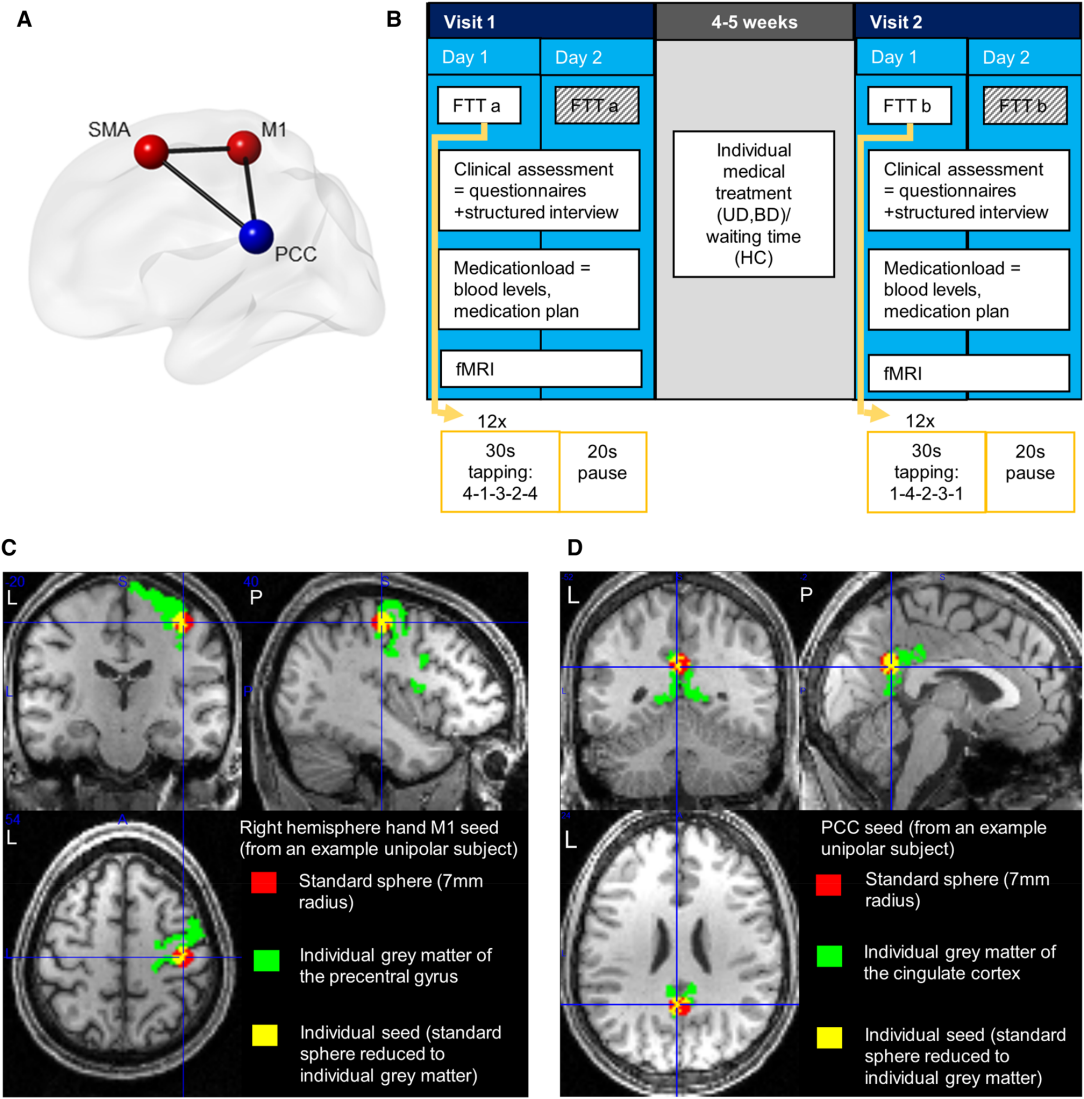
Methods: (A) Connectivity model between regions of interest: supplementary motor area (SMA) and right hemisphere hand primary motor cortex (M1) as part of the sensory motor network (red), posterior cingulate cortex (PCC) as part of the default mode network (blue), (B) Study design: unipolar (UD), bipolar (BD) depressed and healthy control subjects (HC) performed the finger-tapping-task (FTT) on both days of both visits, FTTa and FTT b versions as described in methods section. The data of the second days was not used for the analysis of motor performance. The clinical assessment and the MRI sessions took place on one of the days. The blood sample was taken as close as possible before the MRI measurement, (C) Seed definition technique: exemplification of the overlapping area (yellow) based on FreeSurfer individual segmentation of grey matter (green) and a spherical seed (red) at the MNI coordinates *x* = 40, *y* = − 20, *z* = 54 of functional coordinates for right hemisphere hand motor cortex (M1)^66^ (D) and at *x* = 0 *y* = − 53, *z* = 2 for the posterior cingulate cortex^14^
